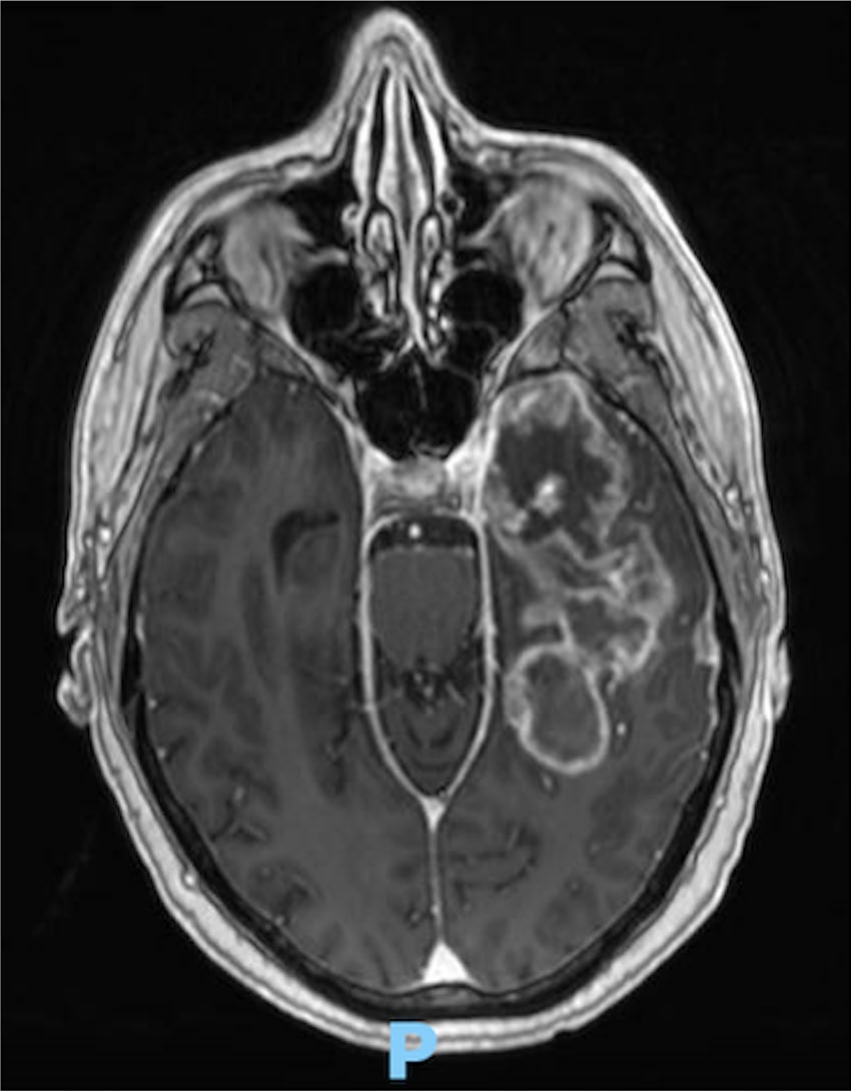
T1-weighted gadolinium-enhanced axial MRI brain demonstrating a high complexity lesion with a 5.7% likelihood of complete resection.
